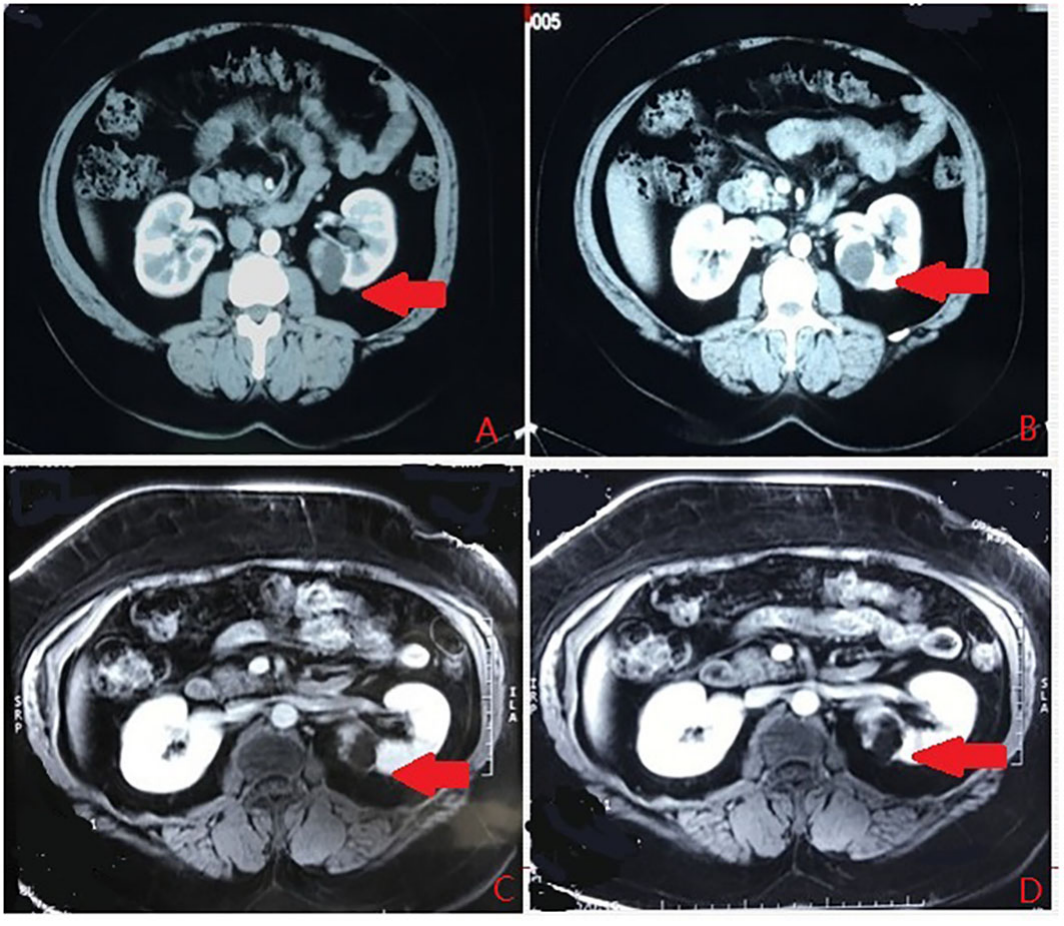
The renal hilum type. (A) Arterial phase. (B) Vein phase. (C) MR image. (D) MR image. The arrows indicates the complex renal cyst.
